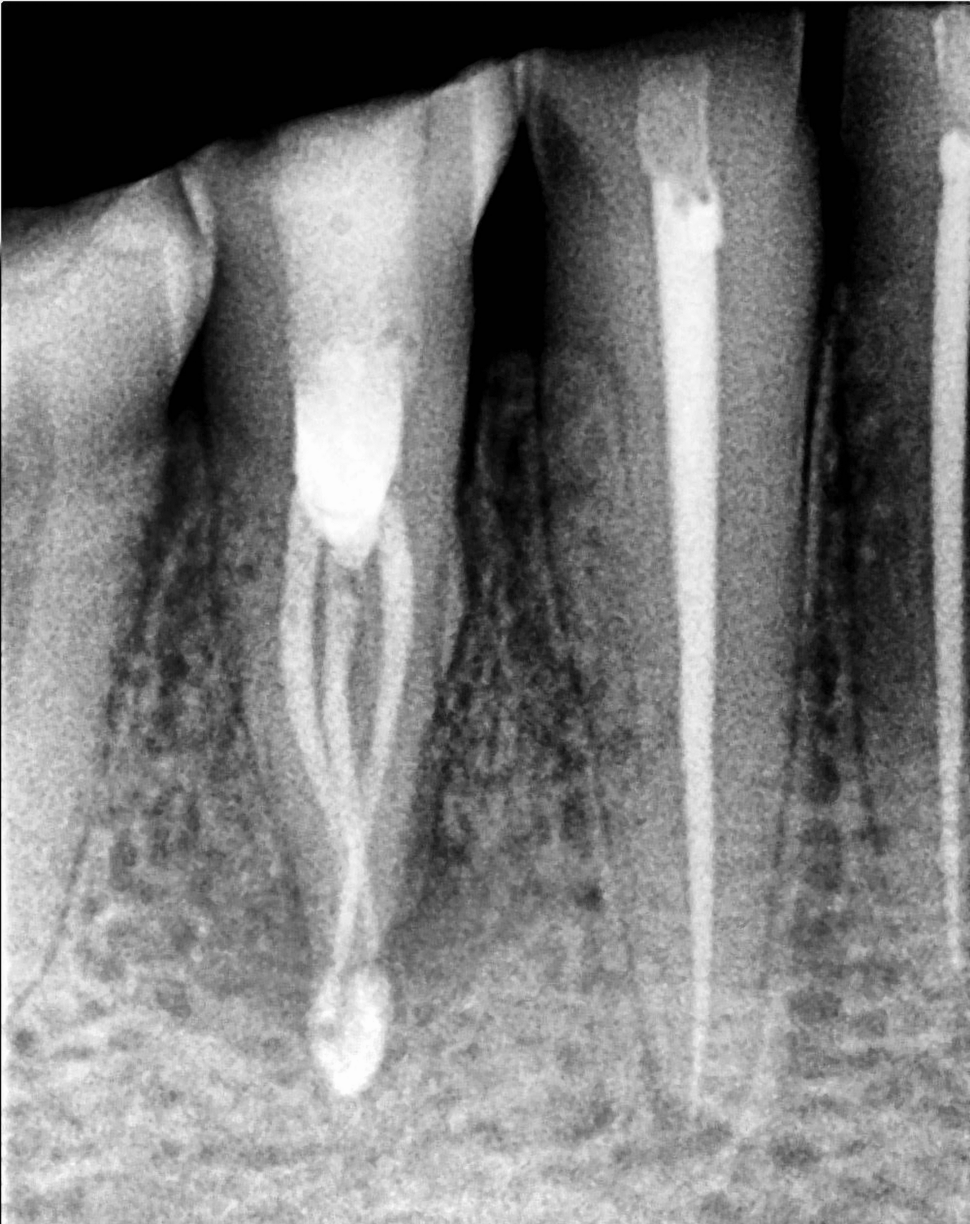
Post-obturation radiograph of the mandibular right first premolar of case 2
